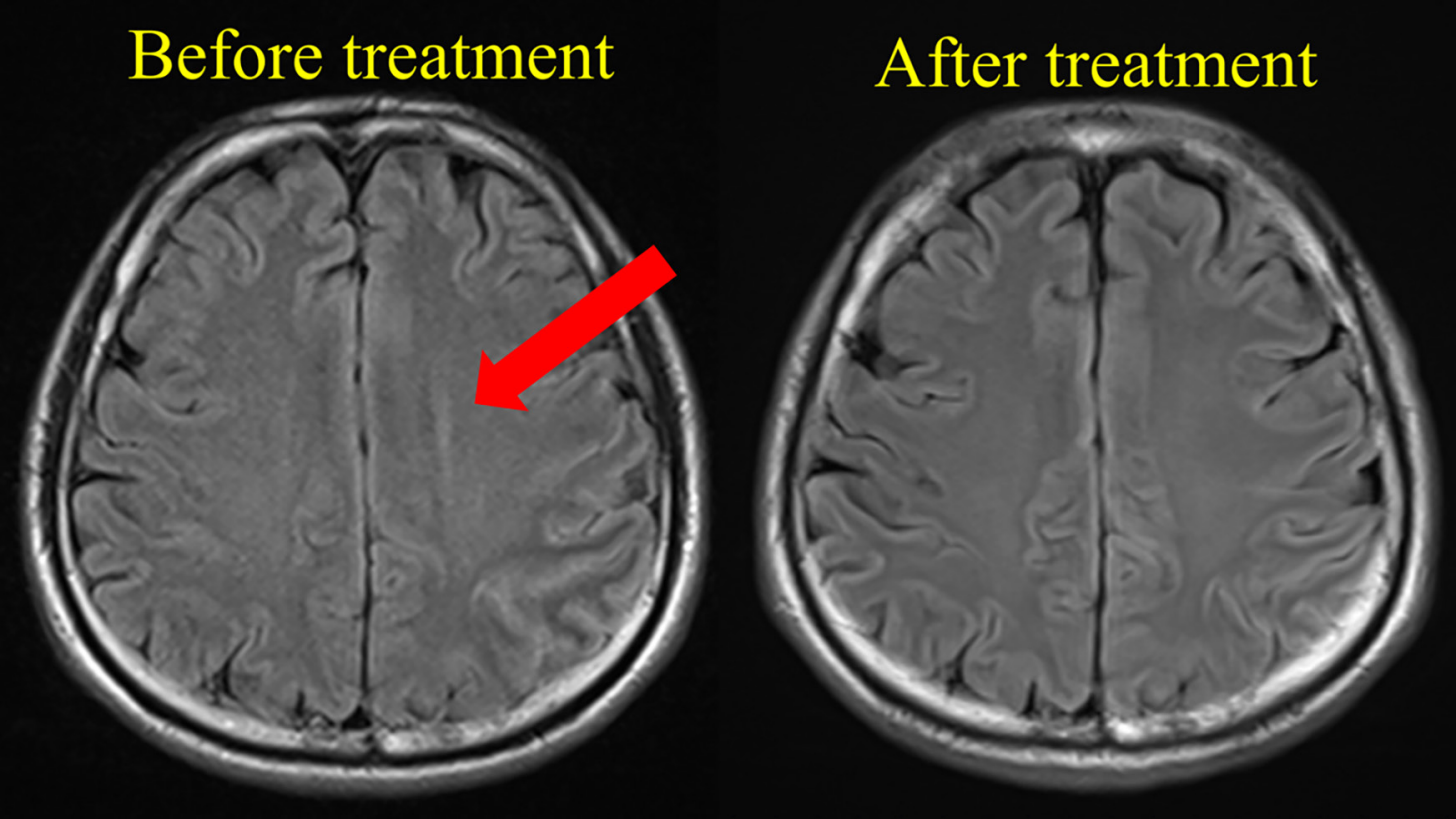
Head magnetic resonance imaging (MRI). MRI images before and after treatment were compared. Before treatment, a high fluid‐attenuated inversion recovery signal intensity was observed in the left parietal cortex. No lesion was observed in the right cerebrum or brainstem.
